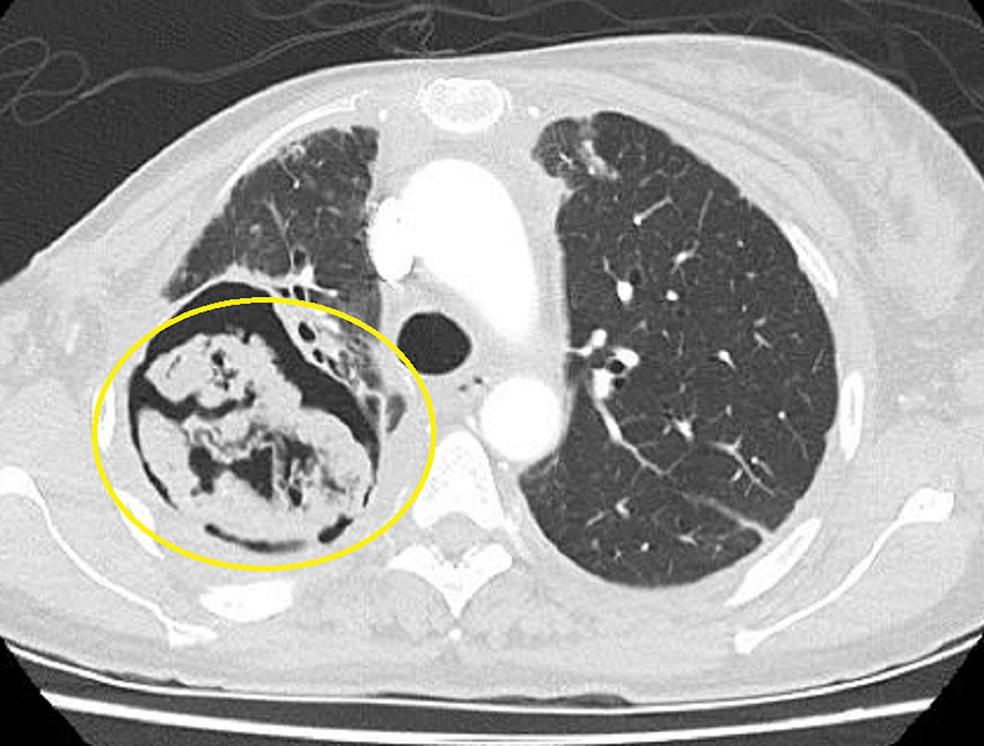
Transverse section of CT scan of the chest showing cavitary lesion on the right upper lobe
